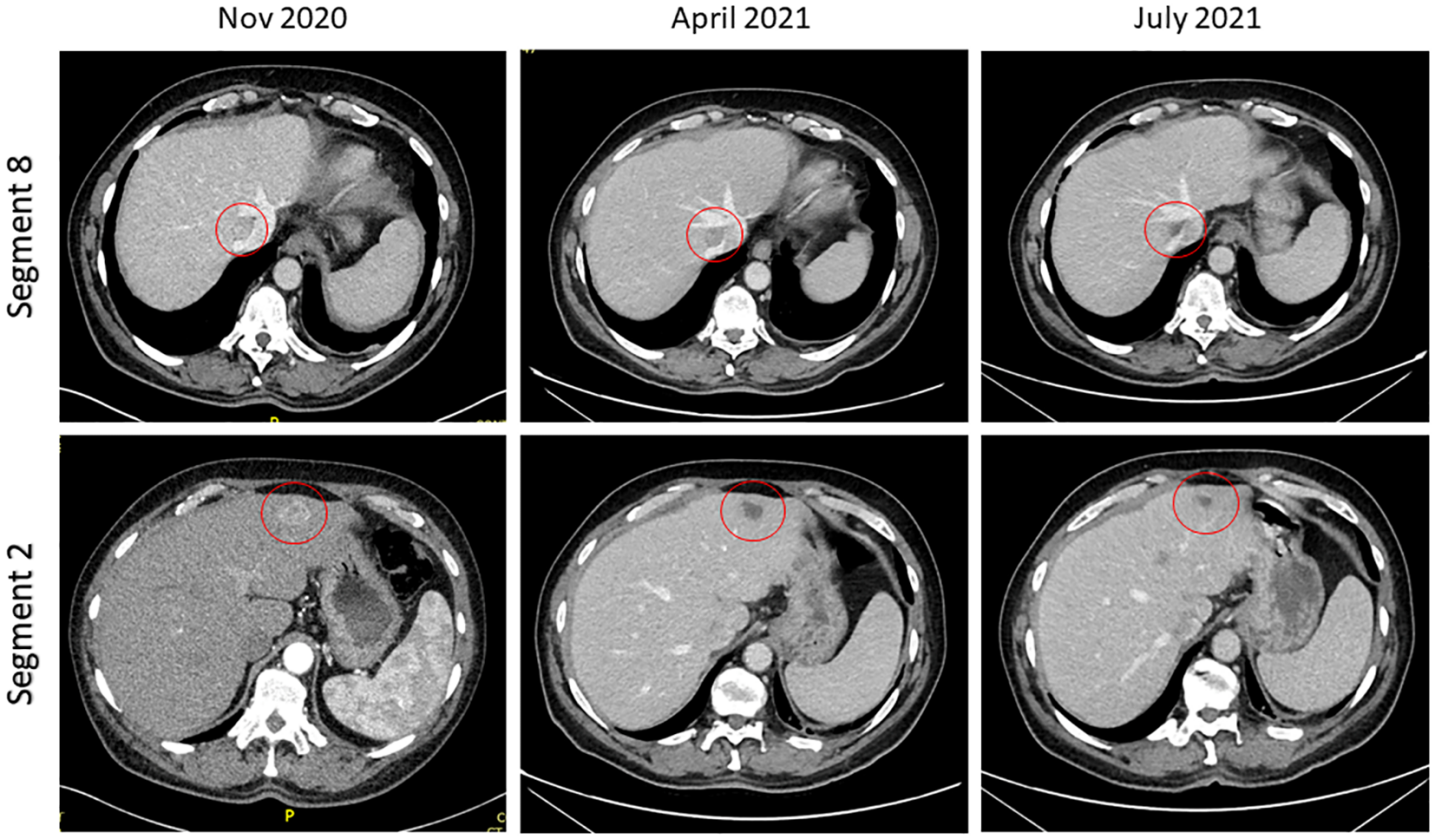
Computed tomography imaging demonstrating response in segment 2 and segment 8 lesions post cycle 4 and post cycle 8 of combination atezolizumab/bevacizumab.
